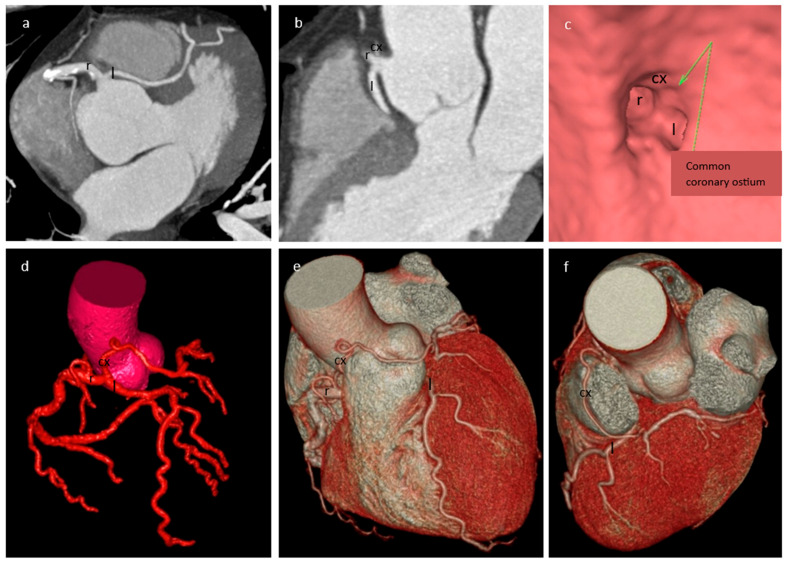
Single coronary artery from the right sinus, with a normal course of the right coronary artery (r), a malignant interarterial course of the left descending artery (l) and a pre-pulmonary course of the left circumflex artery (cx) in three-camber computed tomography images (a,b), virtual endoluminal image (c), and volume-rendering images (d–f).
